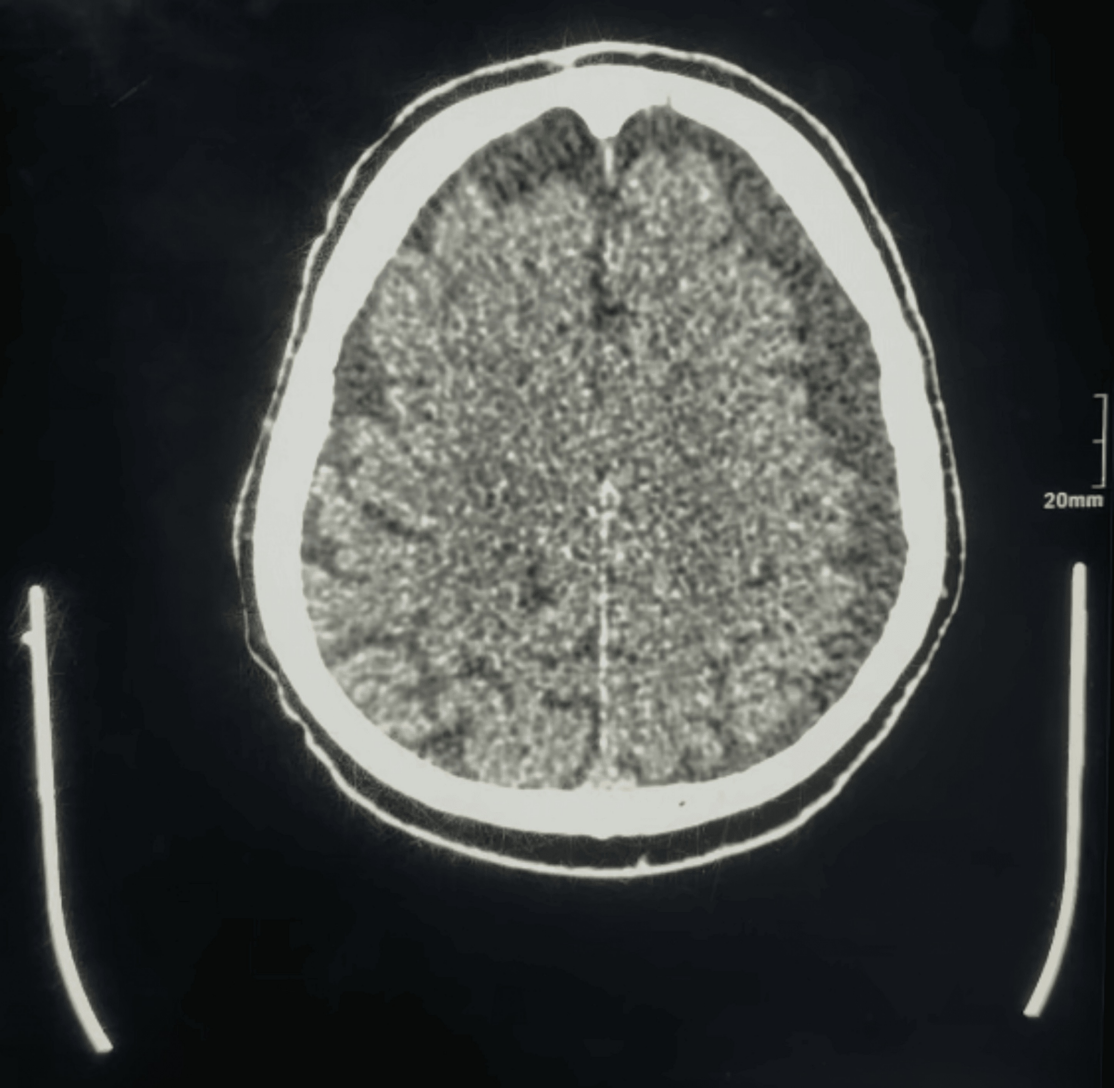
Preoperative sinus CT finding showing the left subdural hygroma CT: computed tomography
